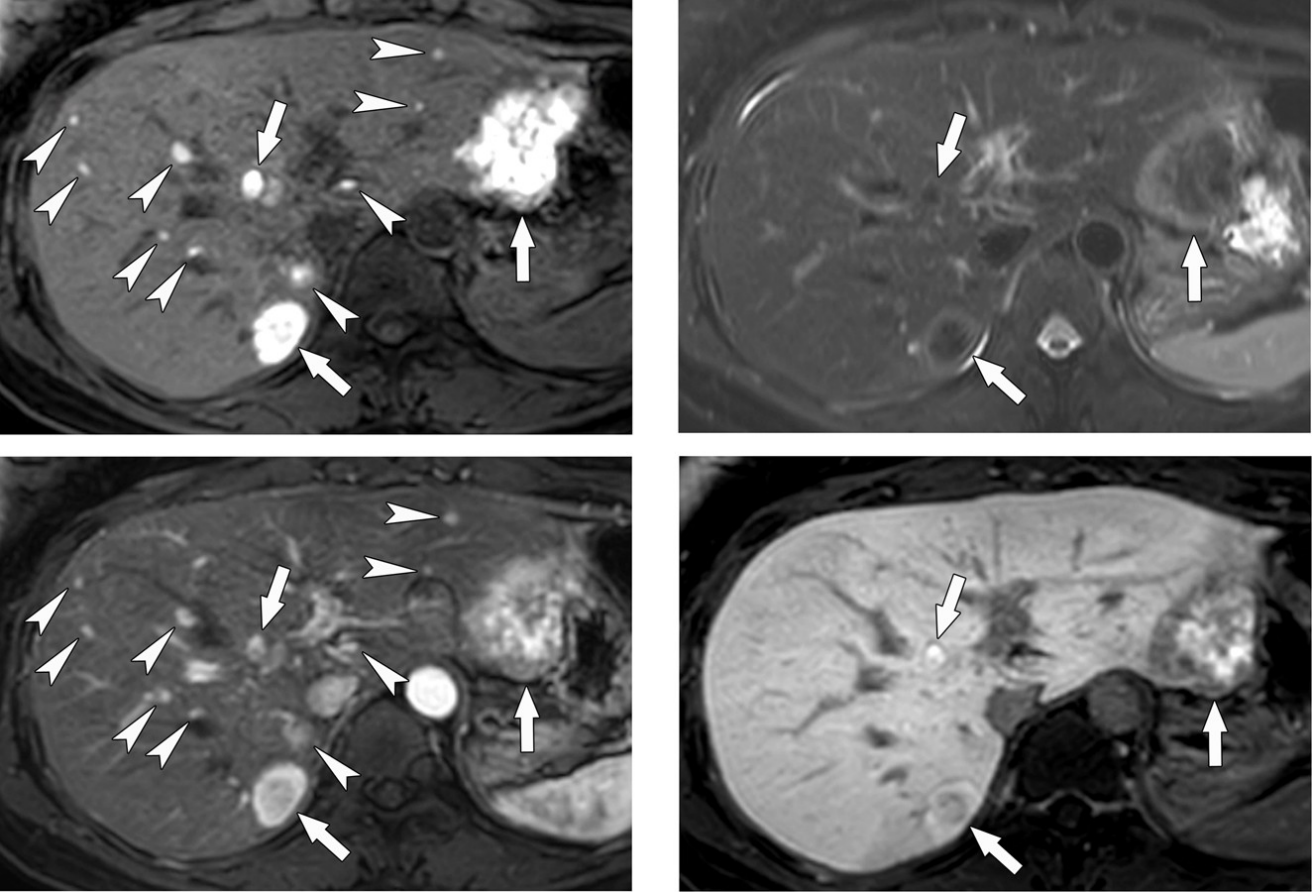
Gadoxetic acid-enhanced MRI in a 53-year-old woman with liver metastases from ocular (choroidal) melanoma. Axial gadoxetic acid-enhanced MR images show multiple hepatic metastases (arrows and arrowheads). Among these lesions, only three metastases are detected (arrows) on a fat-suppressed T2-weighted image (B), and the hepatobiliary phase (D). On precontrast (A) and arterial phase T1-weighted images (C), multiple hyperintense lesions are additionally detected in the liver, suggestive of metastases (arrowheads).
